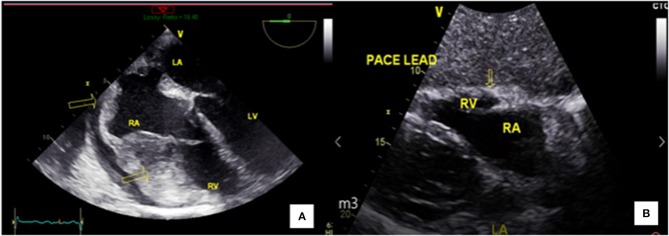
Complete remission after chemotherapy. (A) Transesophageal echocardiography revealing right ventricular infiltration before chemotherapy. (B) Transthoracic echocardiography revealing remission after chemotherapy.
